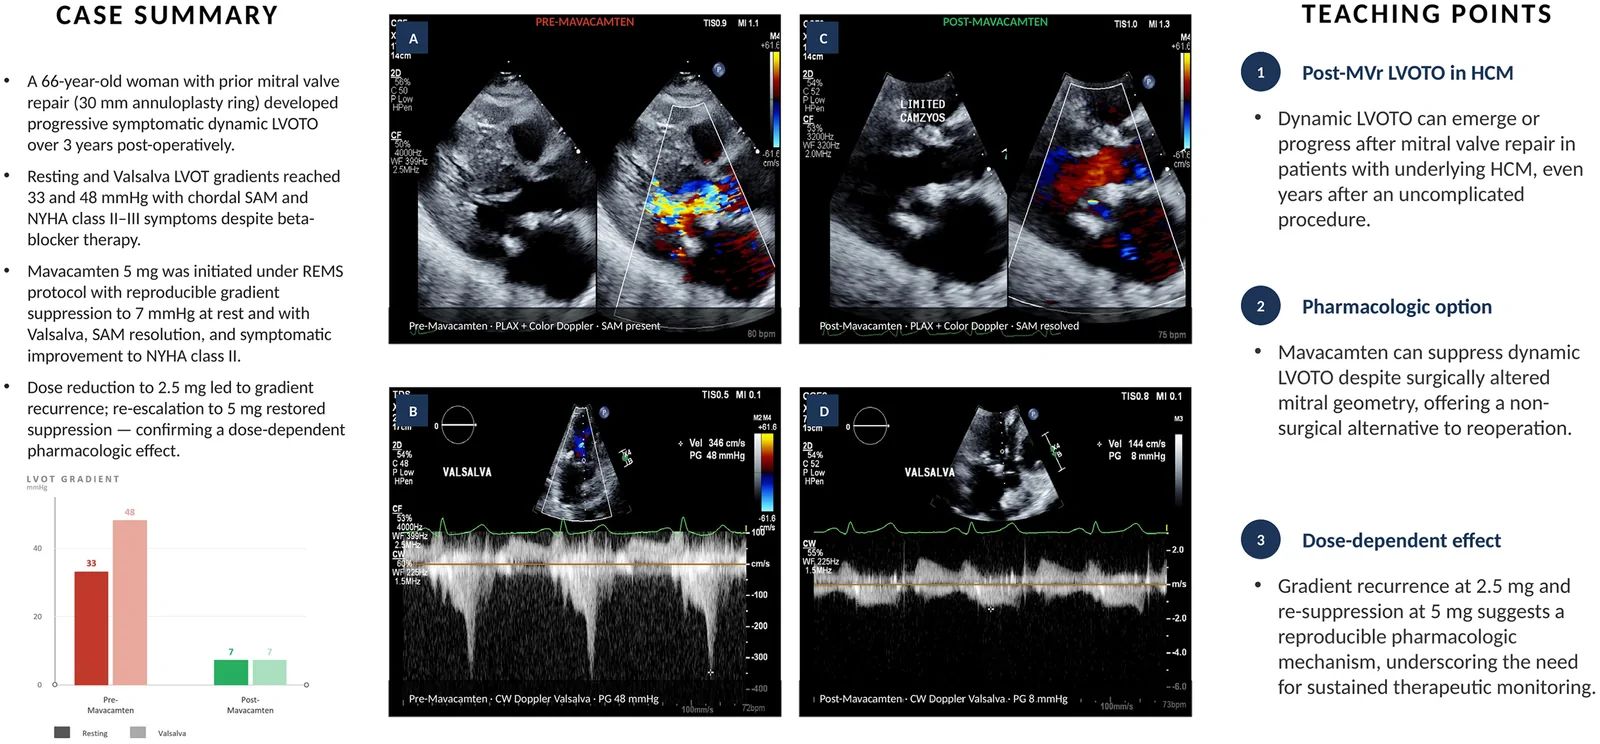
Mavacamten for Dynamic LVOT Obstruction After Mitral Valve Repair in HCM Case summary and key learning points of a patient with dynamic left ventricular outflow tract (LVOT) obstruction following mitral valve repair, treated with a cardiac myosin inhibitor, resulting in significant reduction in LVOT gradient and improvement in symptoms. CW = continuous wave; HCM = hypertrophic cardiomyopathy; LVOTO = left ventricular outflow tract obstruction; MVr = mitral valve repair; PG = peak gradient; PLAX = parasternal long-axis; REMS = Risk Evaluation and Mitigation Strategy; SAM = systolic anterior motion.
